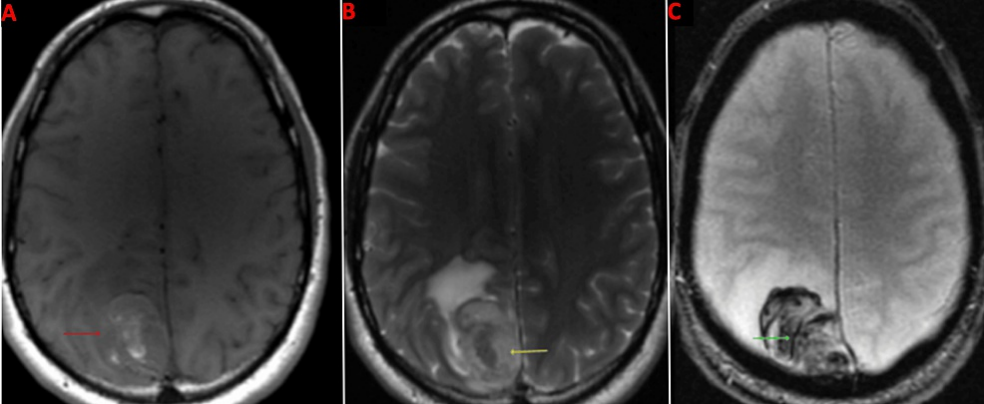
Preoperative MRI brain in the axial plane A: Mass is isointense to the brain on T1 weighted image (red arrow), B: Mass is hyperintense to the brain on T2 weighted image (yellow arrow), C: Mass is hypointense to the brain on GRE (green arrow) sequence GRE: Gradient echo
